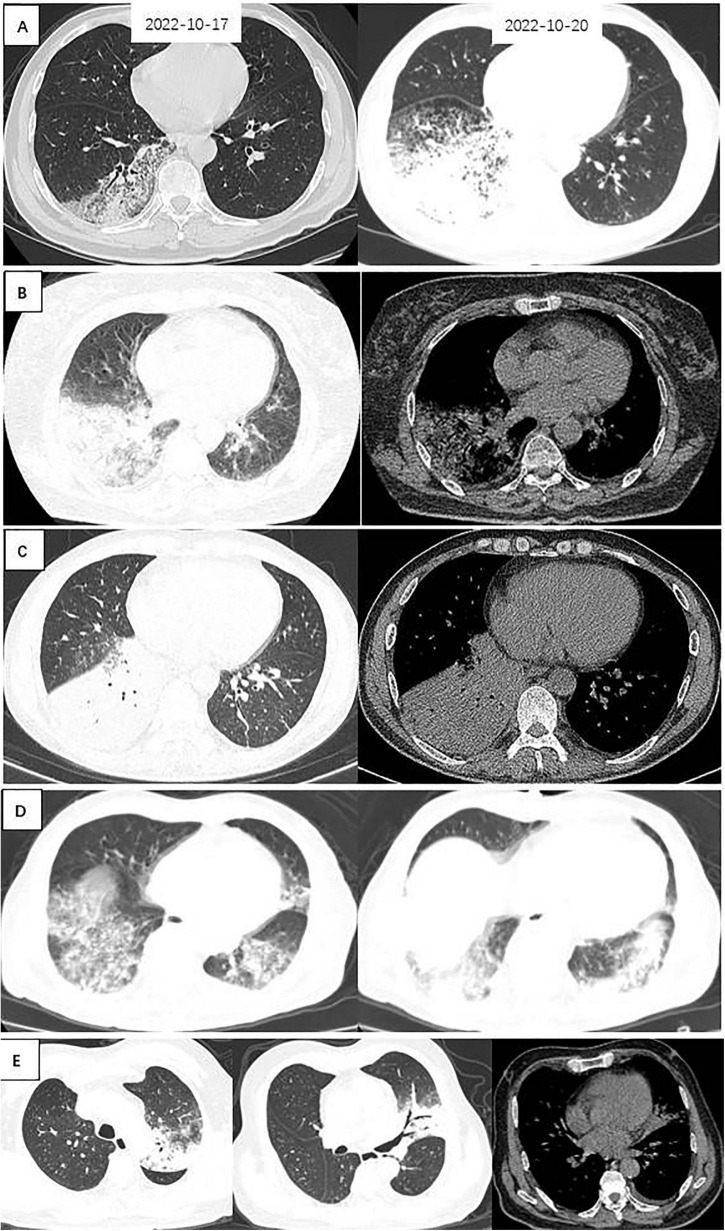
Chest CTs of 5 patients with *Legionella* pneumonia. (A) Patchy ground-glass density, fine latticework shadows with bronchial penetration signs are seen in the right lower lung; a repeat CT 3 days later suggested that the extent of the lesion had increased significantly, and large patches of ground-glass density and fine-mesh shadows were seen throughout the right lower lung, with lamellar solid shadows seen within them. (B) Large solid shadows with bronchial air phases and ground glass density shadows were seen in the right lower lung, with a small amount of pleural effusion. (C) A large solid shadow with bronchial air phase is seen in the right lower lung. (D) Patchy ground-glass densities, fine lattice shadows, and fibrosis are seen in both lower lungs. (E) Left upper lungs exhibit solid shadows, signs of bronchial inflation, ground glass density, and gravity drop.
